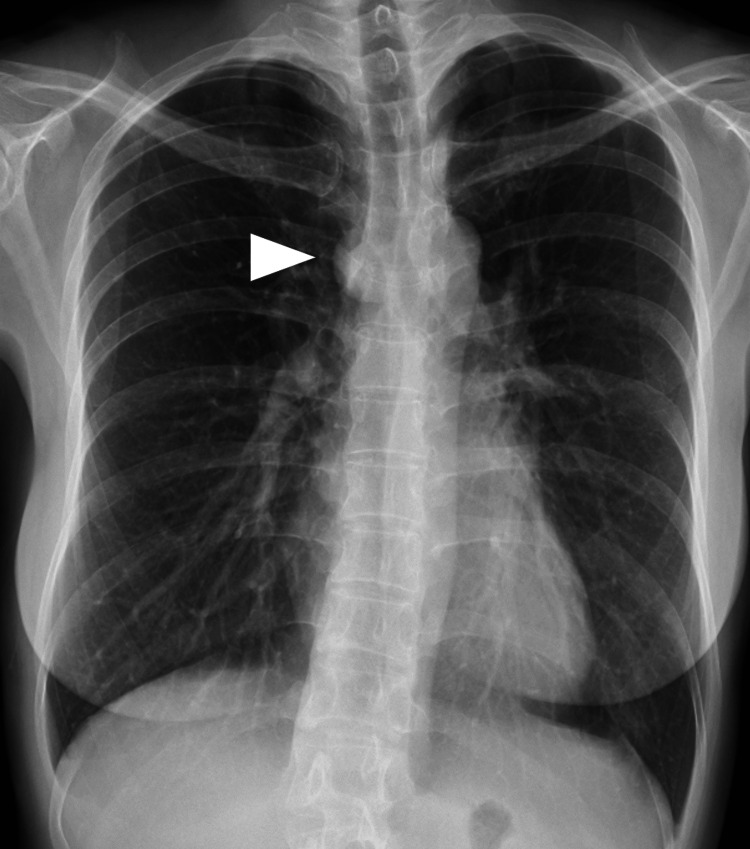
Chest radiography A chest radiograph showing a well-defined nodule in the mediastinum (arrowhead).
